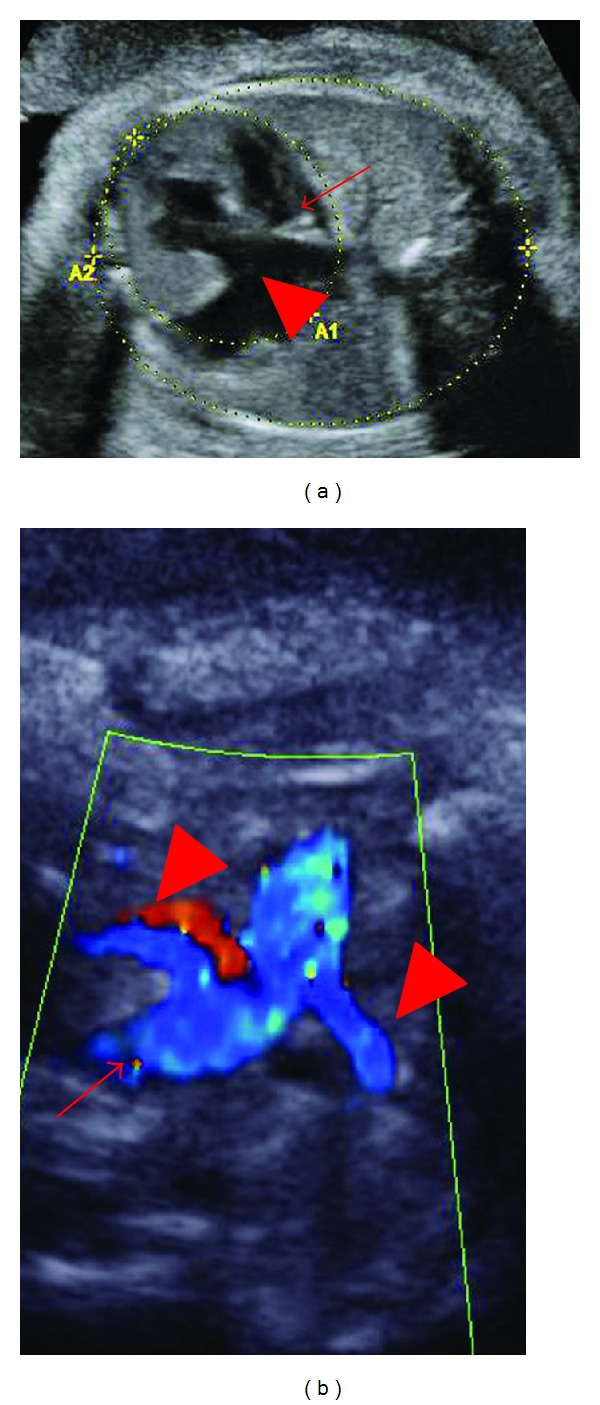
Color Doppler findings of fetal heart anomaly. (a) Ventricular septal defect (arrow):  atrioventricularis communis (arrow head), (b) truncus arteriosus communis where the pulmonary artery branched from the common arterial trunk: truncus arteriosus communis (arrow), pulmonary artery (arrow head).
